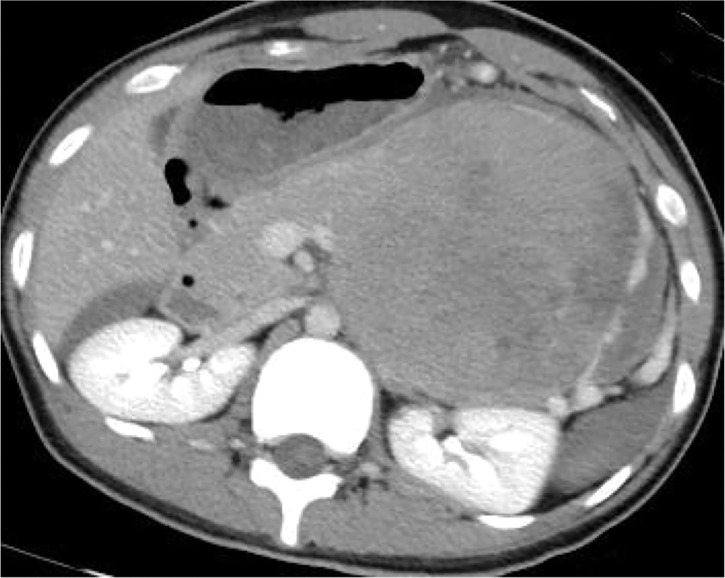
CECT reveals a well-defined 13-cm tumor contiguous with the pancreatic tail. CECT, contrast-enhanced computed tomography
